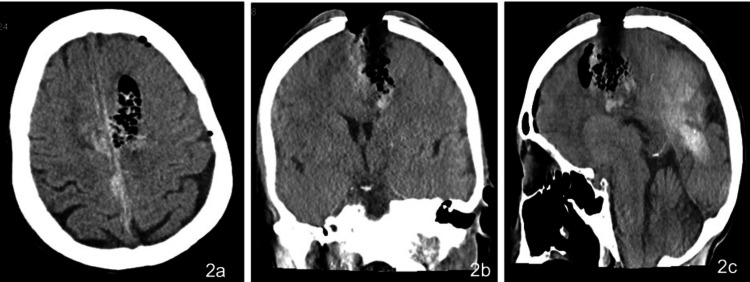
Preoperative (a) axial, (b) coronal, and (c) sagittal plane CT showing open brain injury with surrounding pneumocephalus and foci of infection suggesting cerebral abscess
